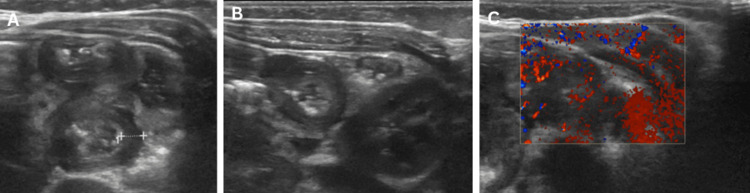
Second episode of ileo-ileal intussusception. (A) and (B) Transverse ultrasound views showing target sign indicating recurrence. (C) Doppler image demonstrating preserved bowel vascularity.
